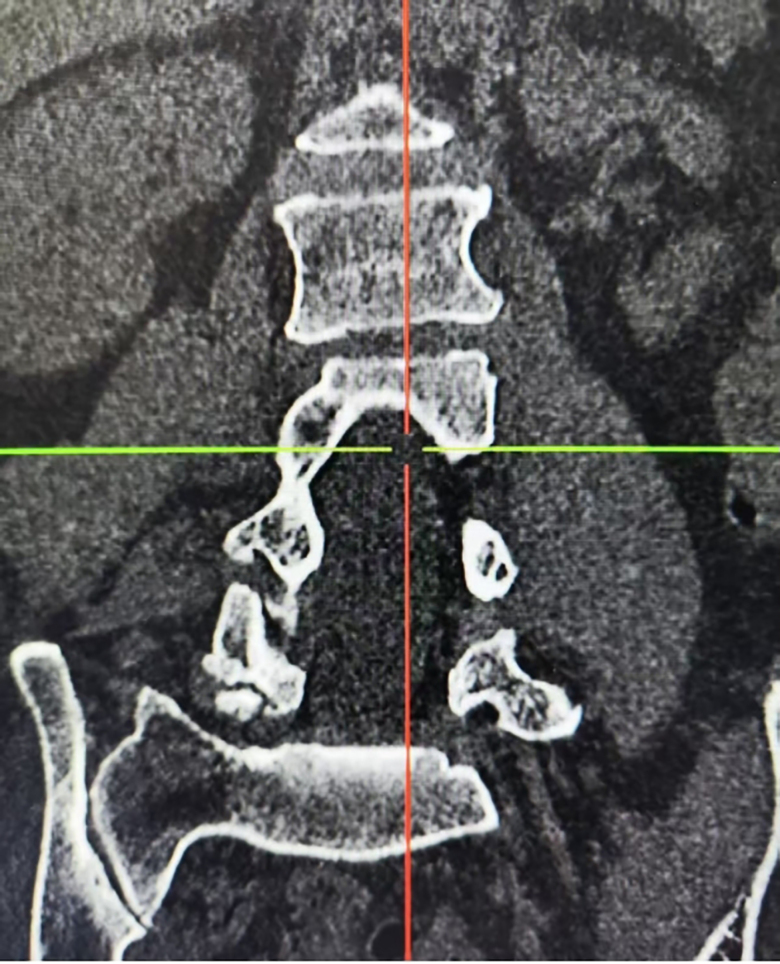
CT plain scan of the entire lumbar vertebrae. CT = computed tomography.
